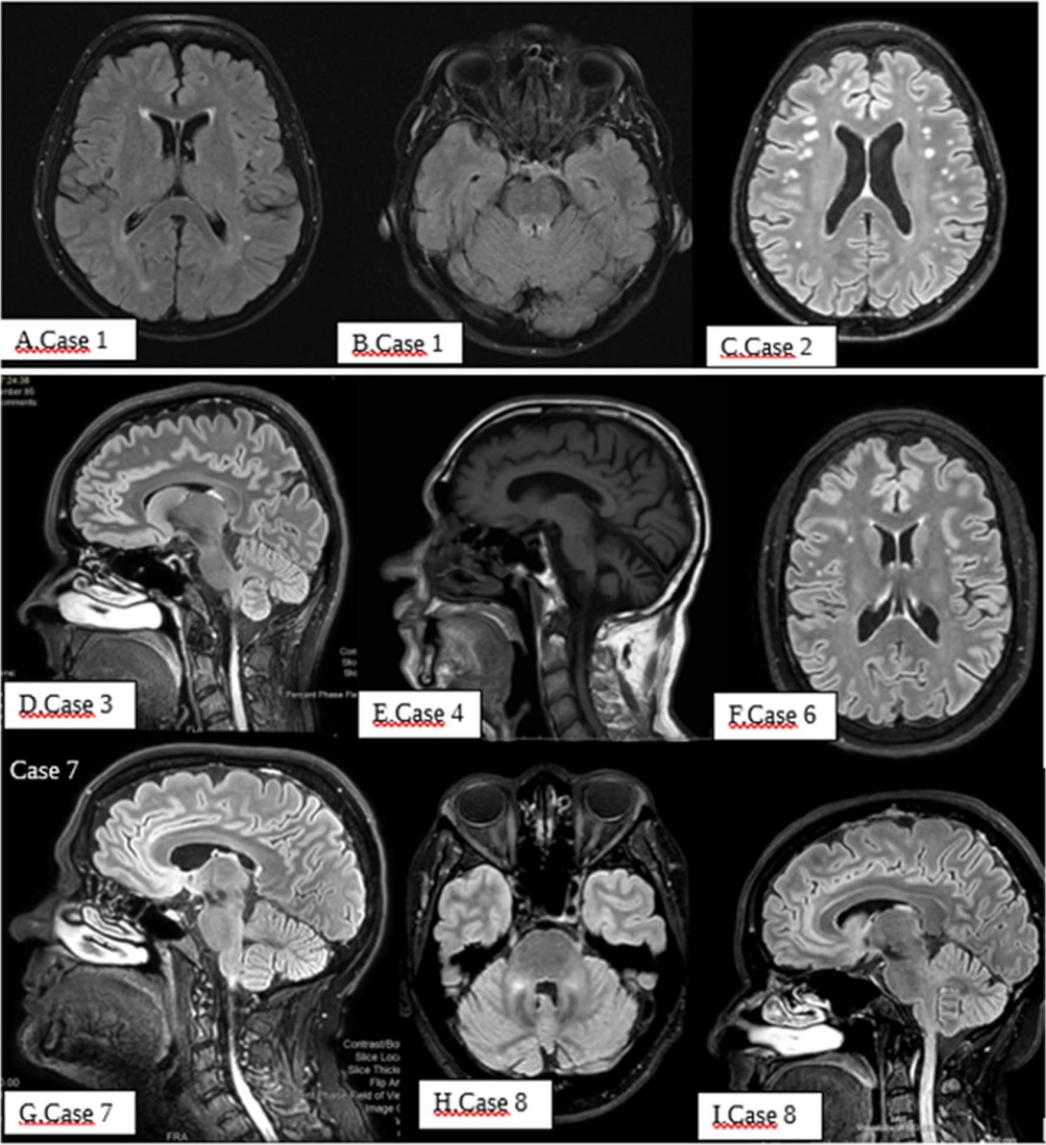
Neuroimaging abnormalities in patients carrying the intermediate alleles. Case 1 (A–B): axial FLAIR-weighted MRI showing hyperintensity of the subcortical white matter (A) and of the superior cerebellar peduncles with extension to the posterior portion of the midbrain (B). Case 2 (C): axial FLAIR-weighted MRI showing multiple subcortical white matter lesions. Case 3 (D): axial FLAIR-weighted MRI showing no alterations. Case 4 (E): sagittal T1-weighted spin echo MRI showing cerebellar atrophy. Case 6 (F): axial FLAIR-weighted MRI showing subcortical white matter lesions. Case 7 (G): sagittal FLAIR-weighted MRI showing mild cerebellar atrophy. Case 8 (H–I): axial (H) and sagittal (I) FLAIR-weighted MRI showing atrophy of the middle cerebellar peduncle and pons
